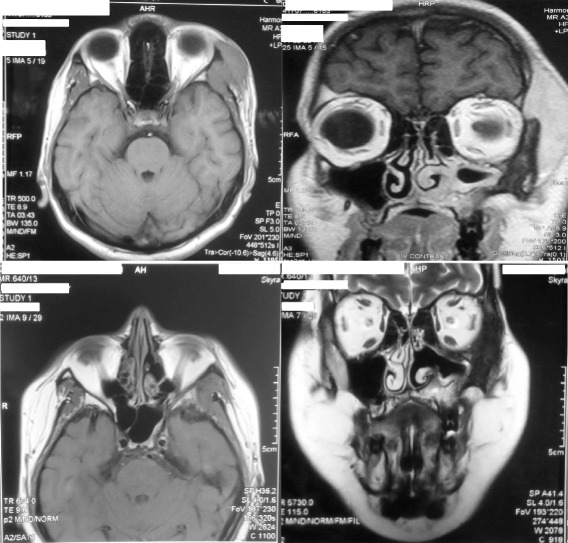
Magnetic resonance images demonstrate the initial appearance and response to glucocorticoid mono-therapy. Top row - Imaging of the head and face revealed abnormal thickening of temporalis. Asymmetry and loss of normal facial planes were seen with infiltrates noted in the left infra temporal fossa. Inflammatory changes are also seen in the ethmoid sinus , left maxillary sinus with muco-periosteal thickening and with non-uniform thickening and sclerosis of sinus wall with erosion postero-laterally. Extension of tumour was noted in the left orbit antero-laterally with lacrimal gland involvement. Bottom row- Imaging demonstrates the initial significant response to steroid monotherapy which prompted rapid tailing off.
